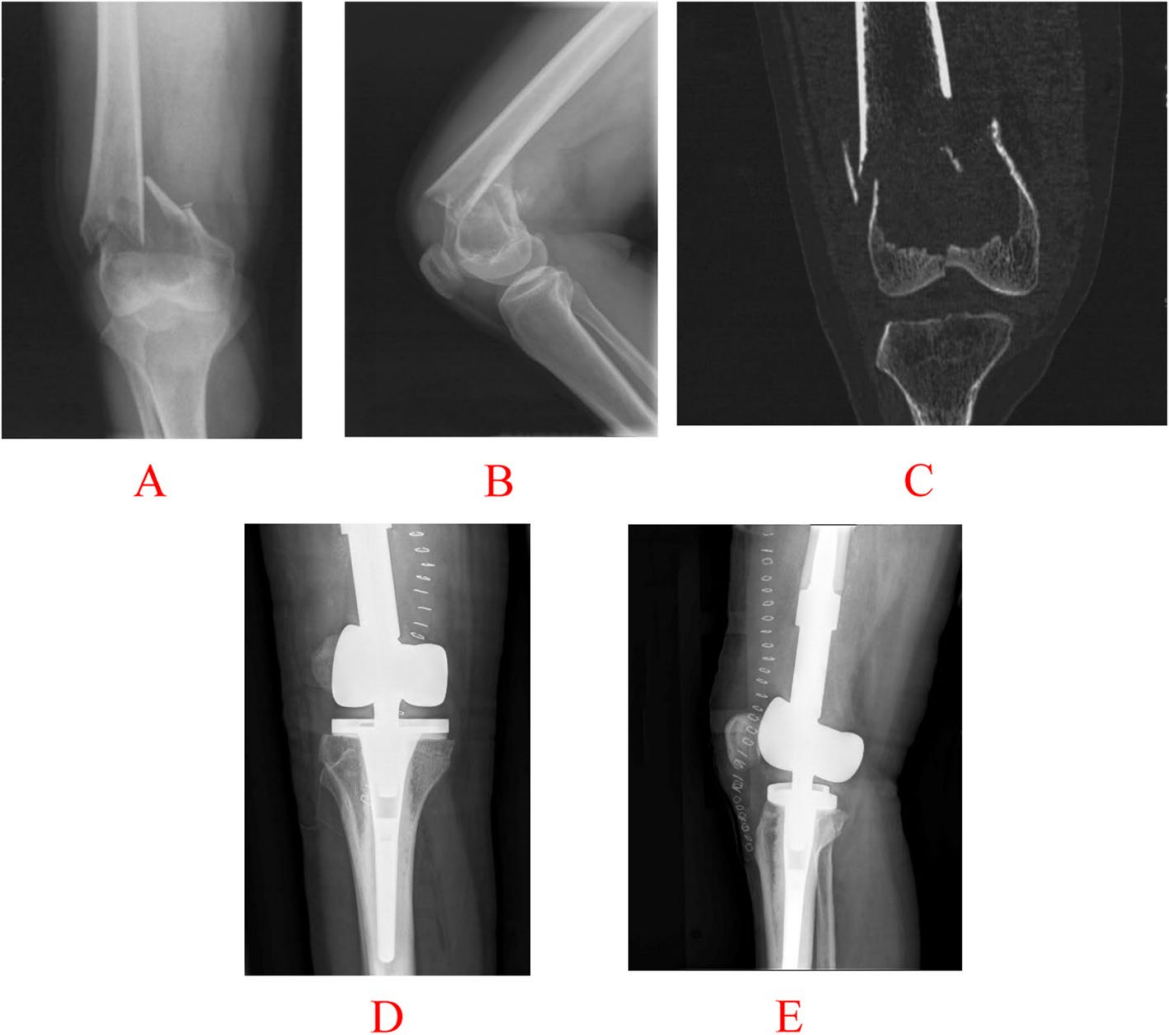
A 39-year-old female with a complex pathological fracture through GCT of the distal femur was treated with resection. A, B Anteroposterior (AP) and lateral preoperative radiographs show bicondylar displaced fracture through a large lytic defect with intraarticular extension. C A coronal CT image shows bone lysis with an absence of the integrity of joint surface, and the distance of fracture displacement is more than 2 mm. D, E AP and lateral postoperative radiographs made immediately after resection reconstructed with prothesis
